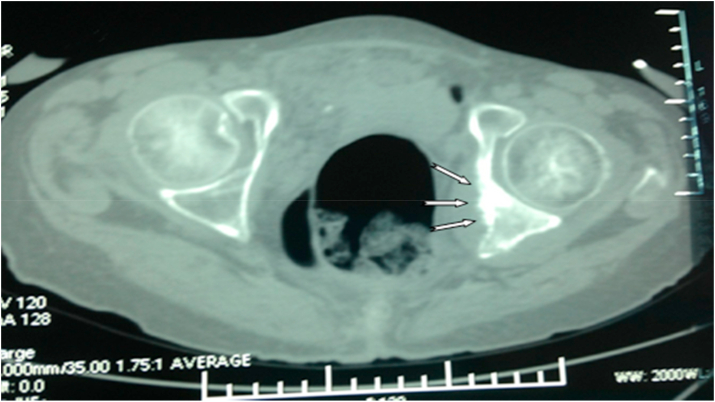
Abdomino-pelvic CT scan showing Bone lesion of the left acetabulum, with reaction of the periosteum burning grass.
